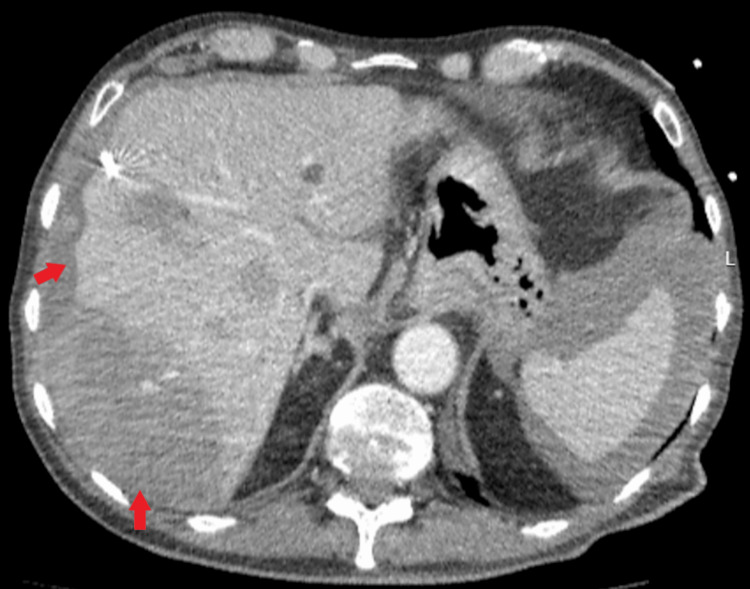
Contrast-enhanced CT abdomen and pelvis showing large right hepatic hematoma on Day 50 There is a large right-sided hepatic hematoma on this portal venous phase study CT: computed tomography
